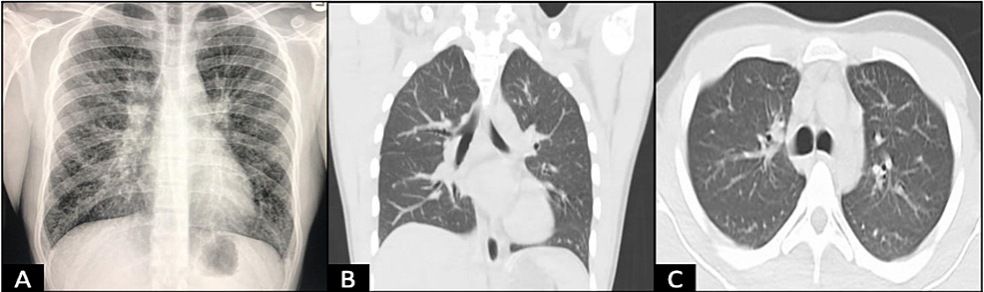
Radiographic findings of allergic bronchopulmonary aspergillosis (A) Posteroanterior (PA) chest radiography demonstrating reticulonodular interstitial markings; (B) coronal and (C) cross-section HRCT chest scans obtained one month after corticosteroid treatment. HRCT: high-resolution computed tomography
